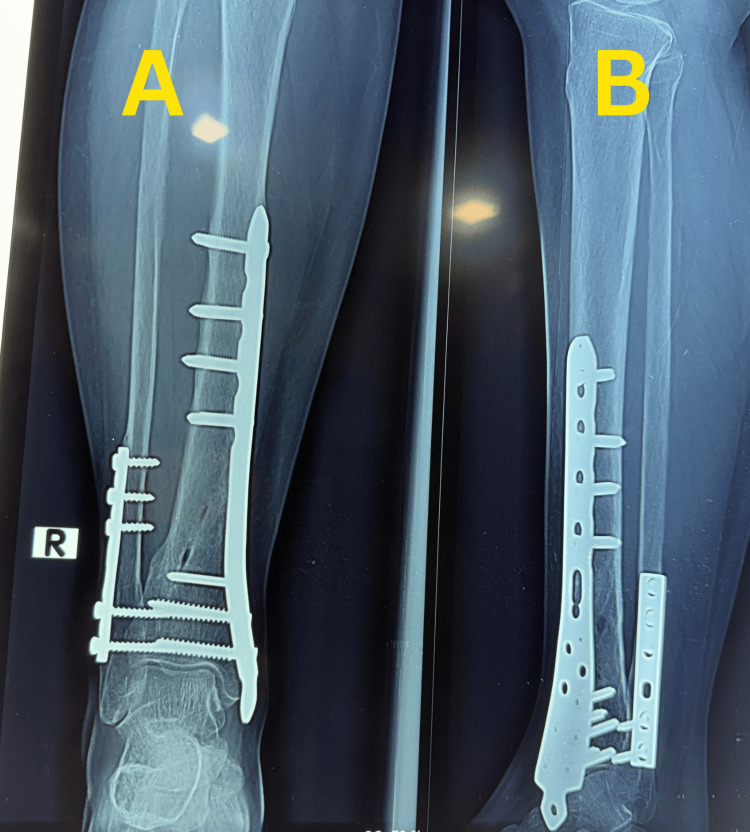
Final follow-up radiographs of Case 2. (A) Anteroposterior view showing complete fracture union with good alignment and remodeling of the distal tibia, with the implant in situ. (B) Lateral view confirming solid union and maintained alignment.
